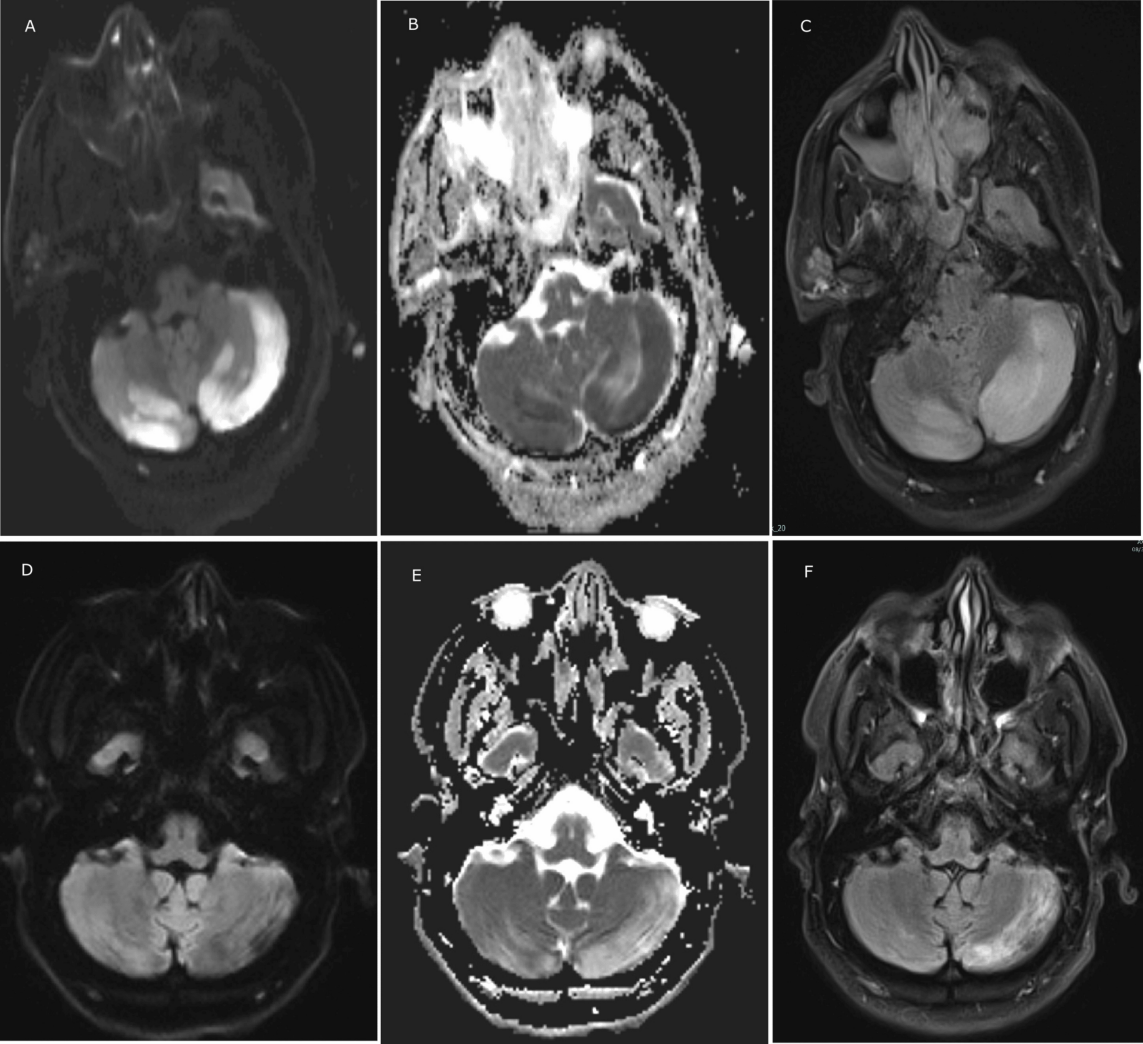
MRI images of the bilateral cerebellum at presentation and at the three-month follow-up ADC/DWI images show diffusion restriction and T2 FLAIR images show edema of the bilateral cerebellum at presentation and at the three-month follow-up A. Diffusion-weighted imaging (DWI) image at presentation; B. Apparent diffusion coefficient (ADC) image at presentation; C. T2 fluid-attenuated inversion recovery (FLAIR) image at presentation; D. DWI image at three months; E. ADC image at three months; F. T2 FLAIR image at three months.
